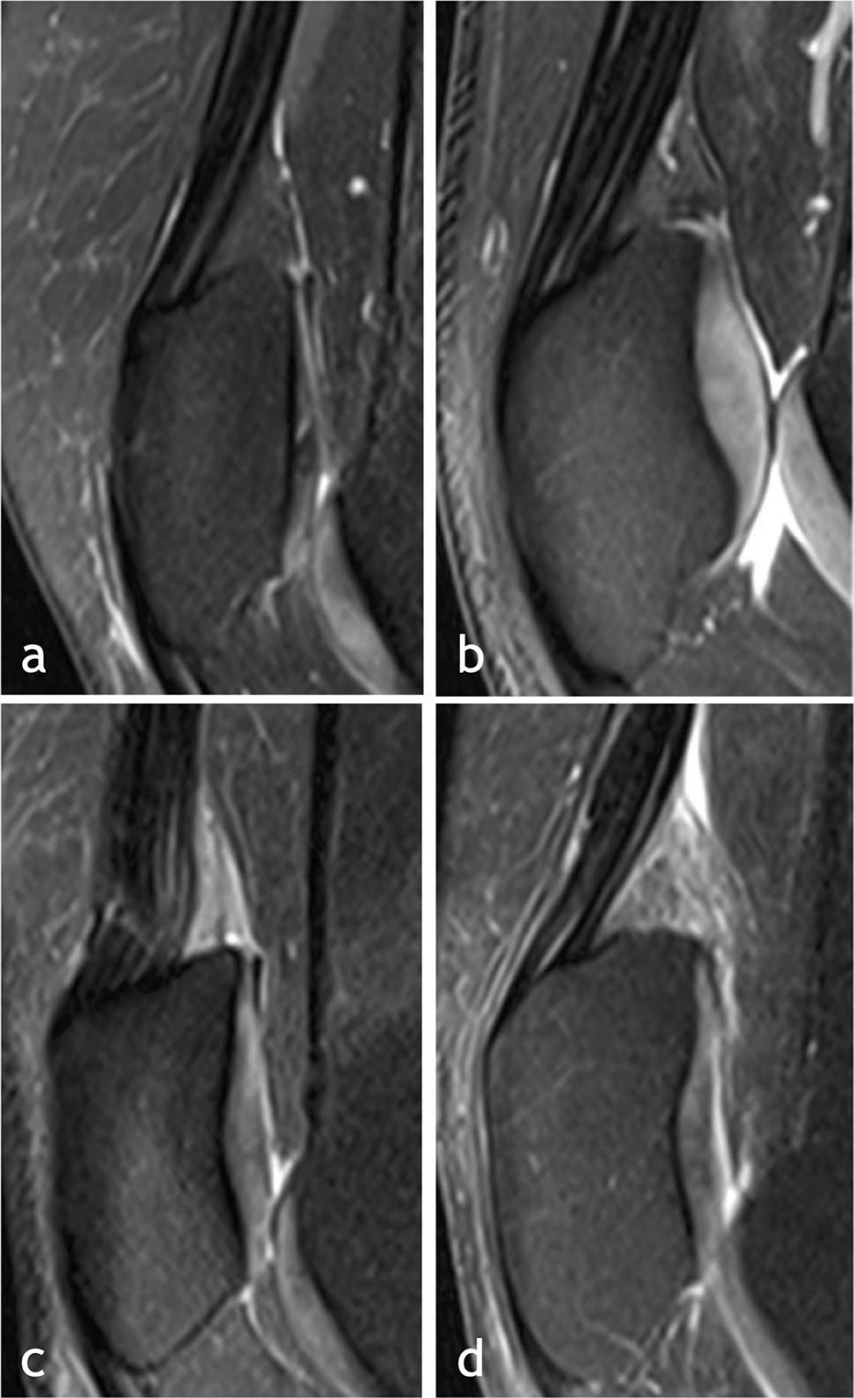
MRI 3 T midsagittal PD TSE fat-suppressed slices showing four different aspects of the suprapatellar fat pad. a No SPFP MRI alteration; b presence of SPFP mass effect only; c presence of SPFP hyperintensity only; and d presence of SPFP hyperintensity and mass effect
